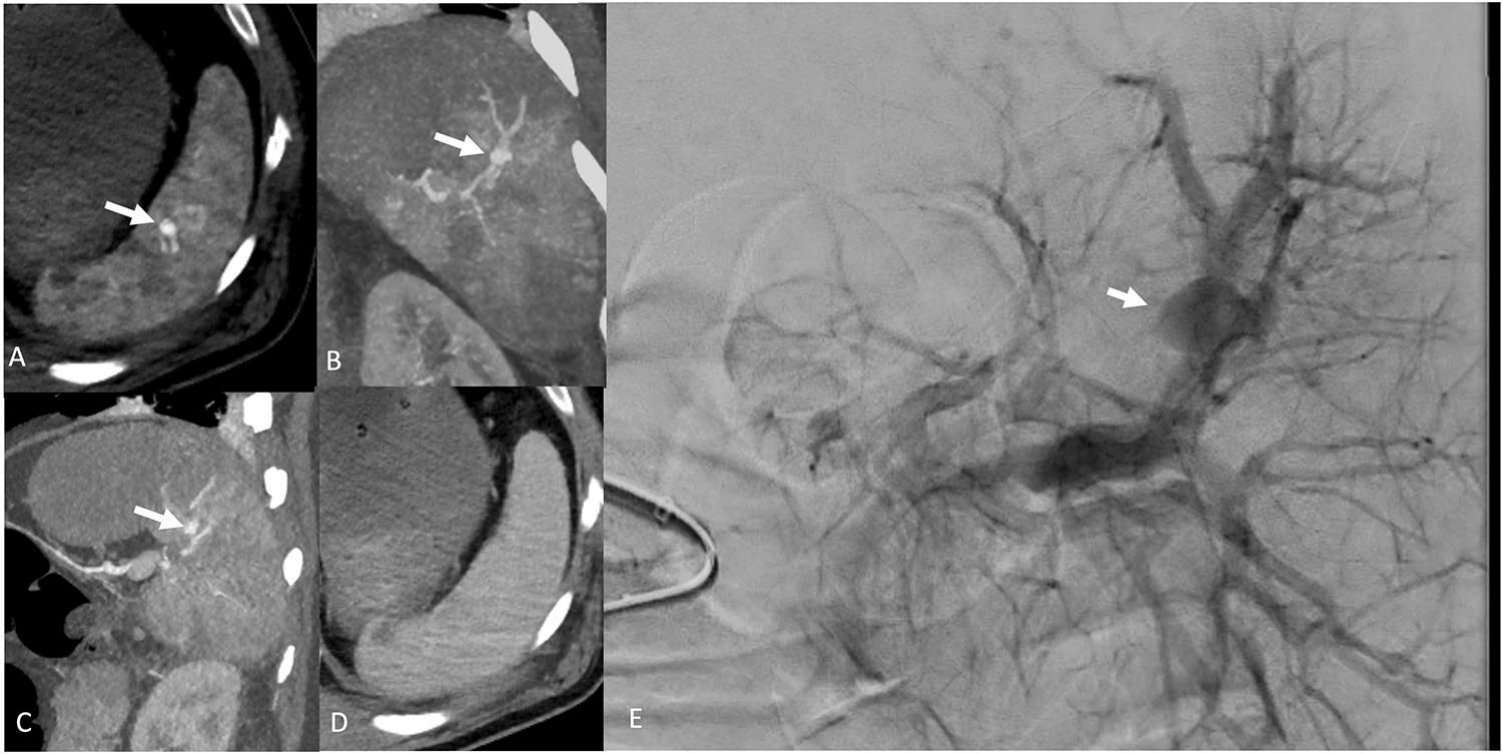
A 55-year-old male involved in a motor vehicle accident with multiple rib fractures and G III kidney injury (not shown), ISS 26. Contrast-enhanced CT scans (A–D). Axial, coronal, and sagittal images in (A–C), arterial and (D) portal venous phases. Images in arterial phase show indistinct area of hyperattenuating foci (arrows). This area washes out at portal venous phase. Patient underwent angiography (E), and contained vascular injury (arrow) was subsequently embolized (not shown).
